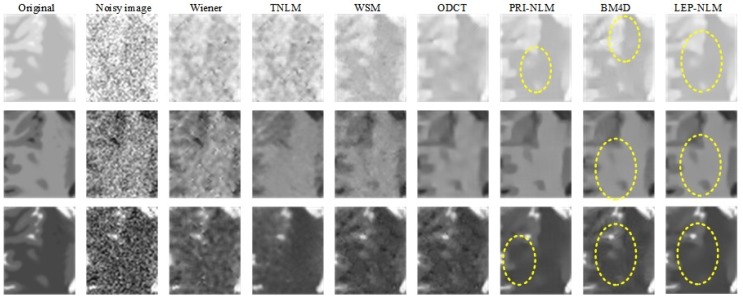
Visual comparison of denoised results for the enlarged ROIs marked with the blue boxes in Figure 4. The top row, middle row, and bottom row are the PD-weighted, T1-weighted, and T2-weighted MR images, respectively.
